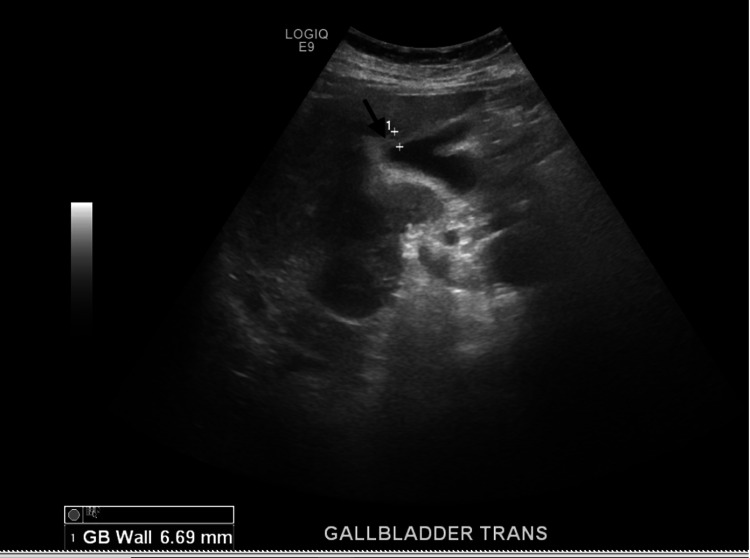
Ultrasound of gallbladder: arrow showing gallbladder wall thickness
